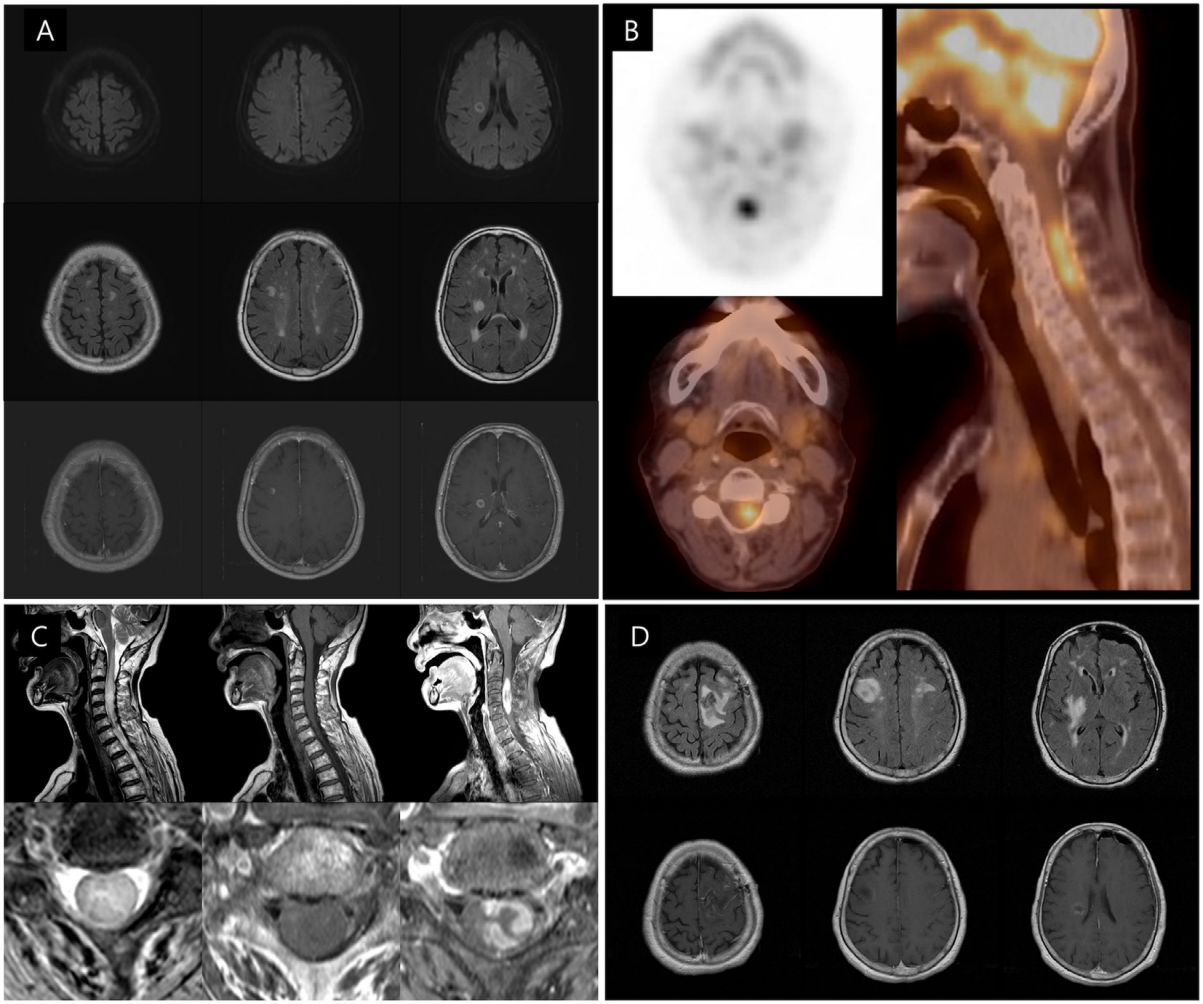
Initial and follow-up brain and spinal cord MRI and F-18 FDG PET/computed tomography (CT) scans of the patient. (A) Diffusion-weighted image (upper row) exhibiting small, round, high, and central low-signal-intensity lesions in the right frontal lobe and internal capsule. T2 fluid attenuation inversion recovery (FLAIR) image (middle row) showing multiple small high-signal-intensity lesions in both cerebral hemispheres. Enhanced T1-weighted image (lower row) demonstrating rim enhancement in both the frontal cortical and internal capsular lesions. (B) F-18 FDG PET/CT scan showing another high-uptake lesion in the patient’s neck. (C) The cervical spinal T2 FLAIR image revealing high-signal-intensity lesions with surrounding edema at the C2–C6 level of the spinal cord, especially the left pyramidal tract. The cervical spinal enhanced T1-weighted image displaying heterogenous enhancement, at the same level. (D) The follow-up T2 FLAIR and enhanced T1-weighted images performed after biopsy showing that both frontal cortical and subcortical high-signal-intensity lesions have increased in size.
